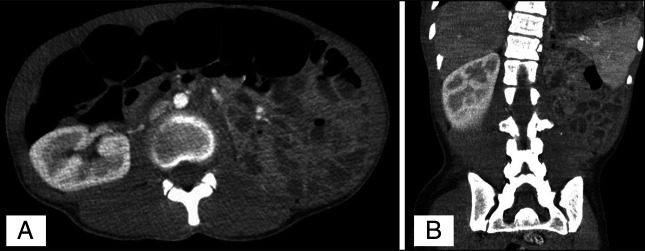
Abdominal and pelvic computed tomography axial (A) and coronal (B) demonstrating resolution of small bowel intussusception.
